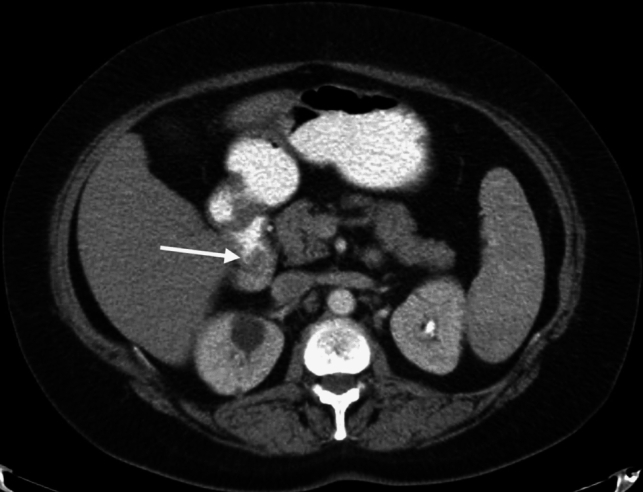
Axial IV contrast-enhanced CT image of a 39-year-old female patient showing a well-defined, isodense filling defect (arrow), diagnosed as an epithelial polyp
